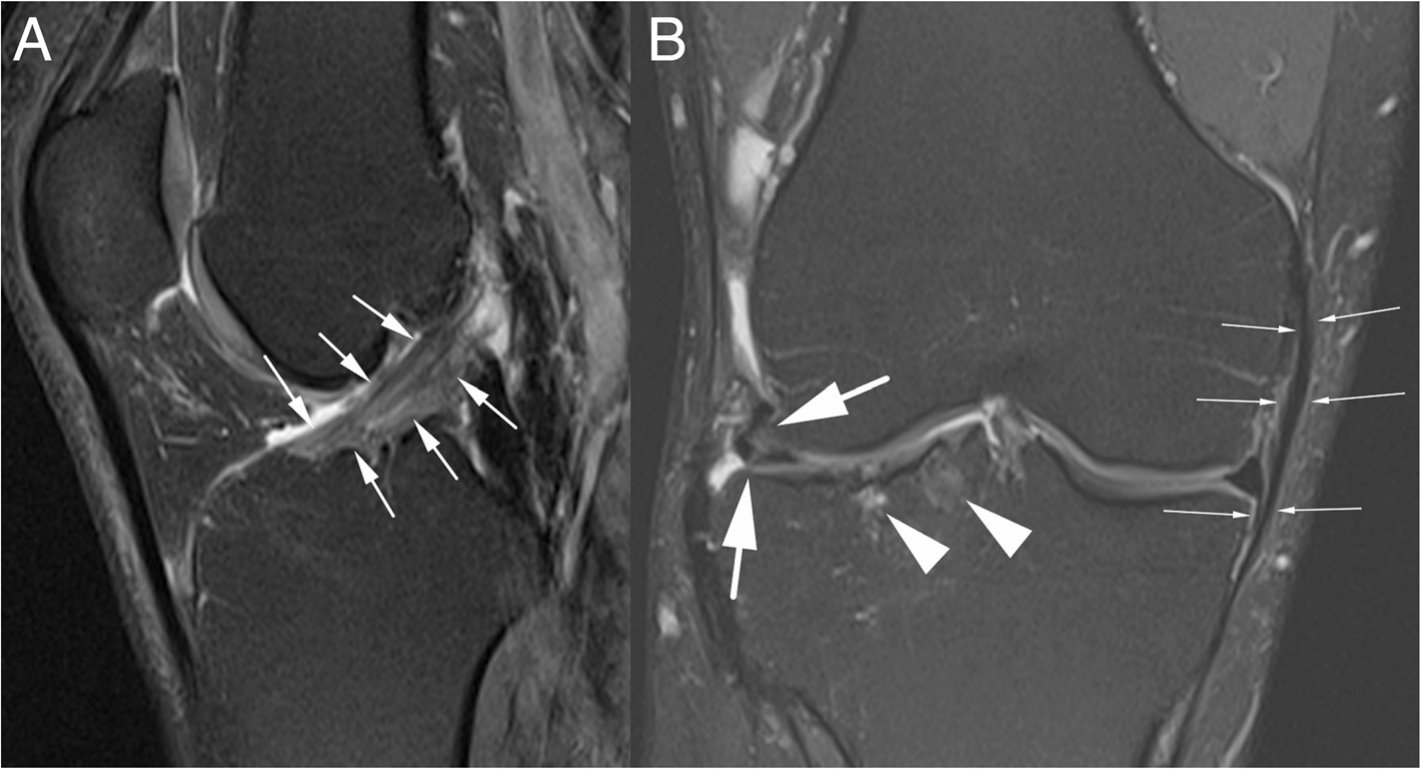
Fat-suppressed proton-density-weighted (FS-PDw) magnetic resonance imaging (MRI). Fig. 5
